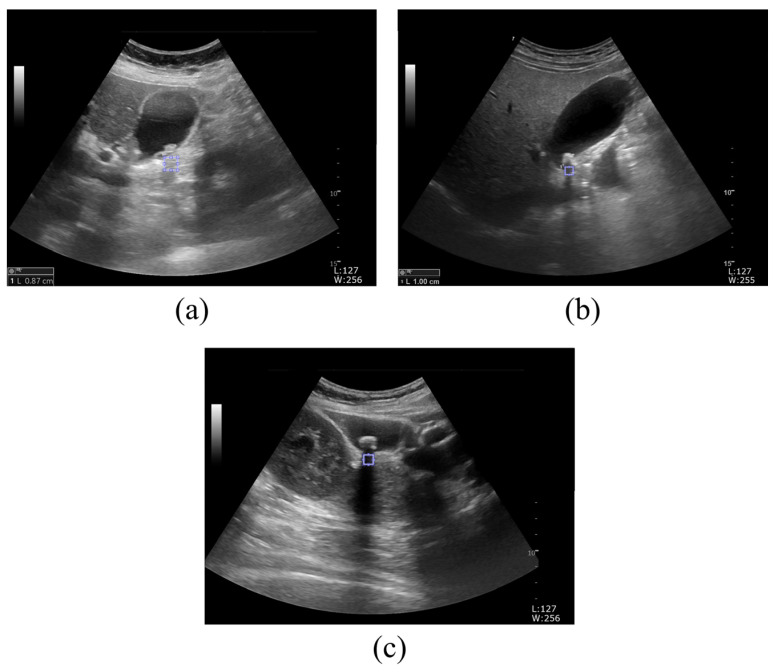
Gallbladder stone ultrasound images acquired for GLCM analysis. Sample ultrasound images of suspected gallbladder stones, with probabilities of (a) 50–60%, (b) 60–80%, and (c) ≥80%, analyzed by doctors and sonographers.
